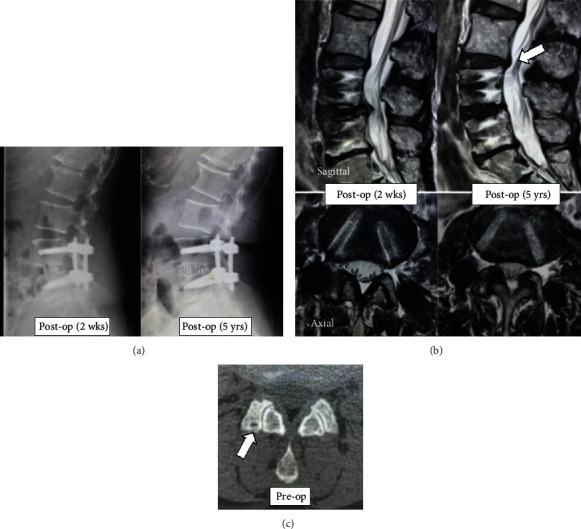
Representative images from the case of a 61-year-old female with L4 spondylolisthesis. (a) Postoperative lateral radiographs at 2 weeks and 5 years after surgery. Posterior slippage of the L3 vertebra and decrease in L3/4 disc space height were found. (b) Sagittal (upper panel) and axial (lower panel) MRI images at L3/4 disc level at 2 weeks and 5 years after surgery. Posterior protrusion of the L3/4 disc (white arrow) is present at 5 years postoperatively. The axial view shows a decrease in the dural sac cross-sectional area and thickening of connective tissue and ligaments around the facets at 5-year follow-up. (c) Preoperative CT axial view at the L3/4 facet joint shows overall joint space narrowing and a subchondral cyst (white arrow).
